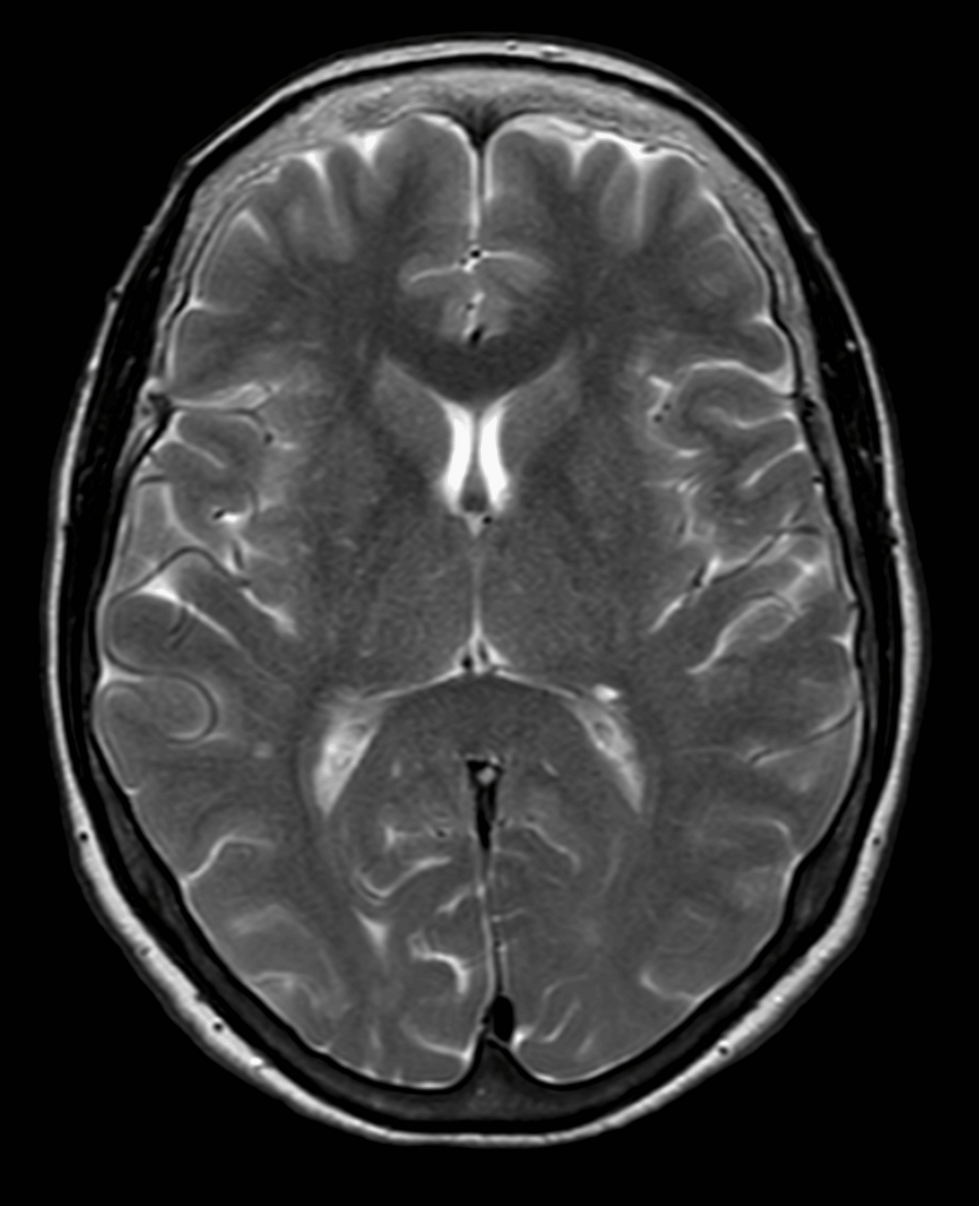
MRI brain with no evidence of an acute infarct or any other significant abnormality
